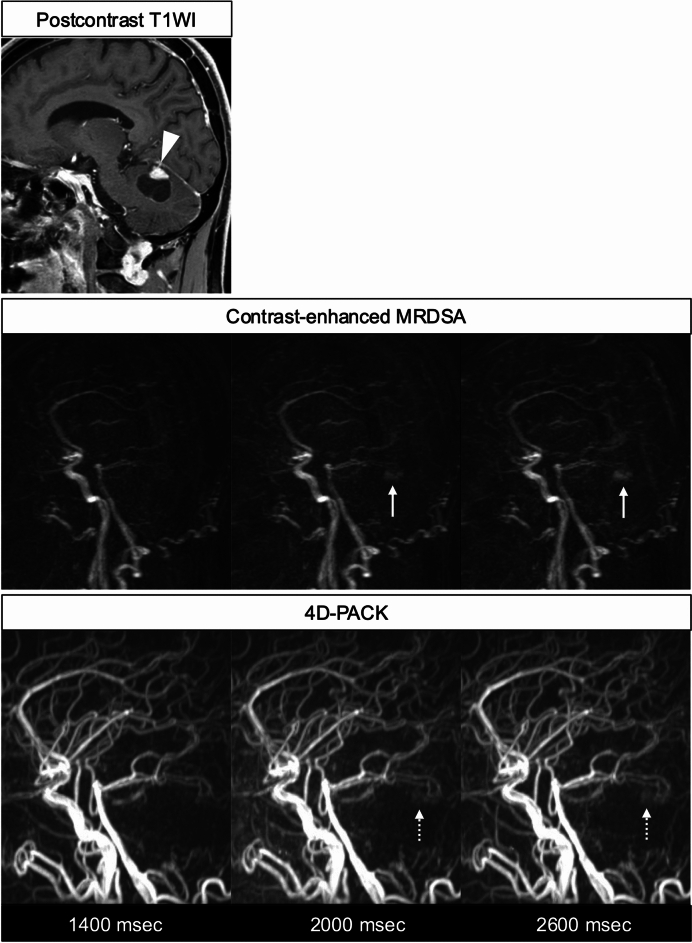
Hemangioblastoma. The postcontrast T1-weighted image (T1WI), contrast-enhanced MRDSA, and 4D-PACK are shown from the upper to the lower columns in that order. The postcontrast T1WI shows a cystic tumor with a mural nodule in the cerebellum (arrowhead). Contrast-enhanced MRDSA exhibits tumor enhancement corresponding to the nodule from the early arterial phases (arrows). 4D-PACK demonstrates a high signal that corresponds to the mural nodule, similar to contrast-enhanced MRDSA (dotted arrows). 4D-PACK: 4D-MR angiography based on pseudo-continuous arterial spin labeling combined with CENTRA-keyhole and view-sharing, MRDSA: magnetic resonance digital subtraction angiography
